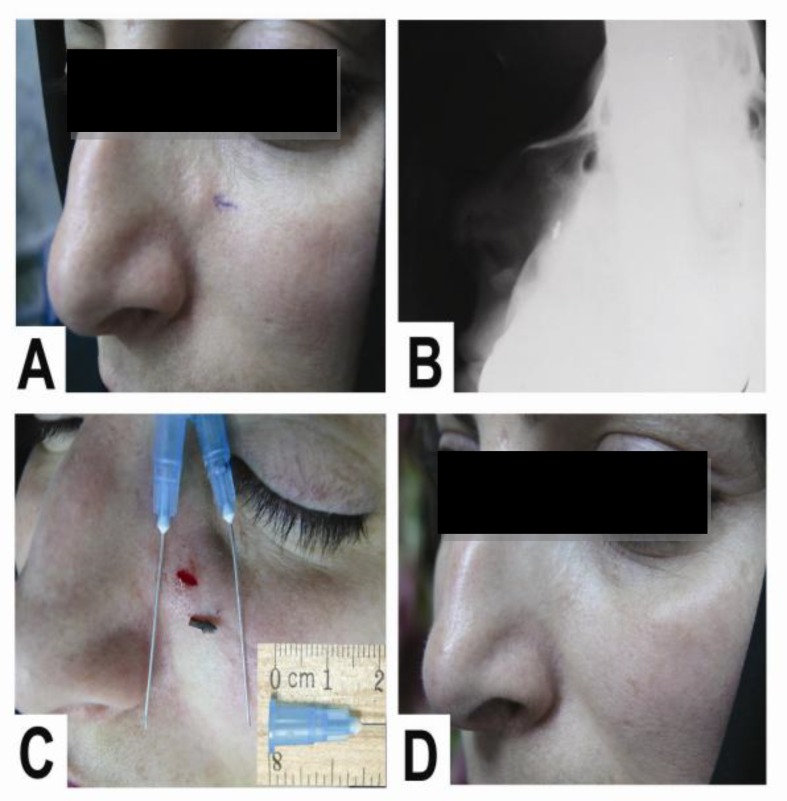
A, 32-year old woman injured 20 years earlier; B, The neglected pen tip as observed radiographically; C, Localization and removal of the pen tip via a small incision; D, Six months after surgery. Note the mild scar formation
